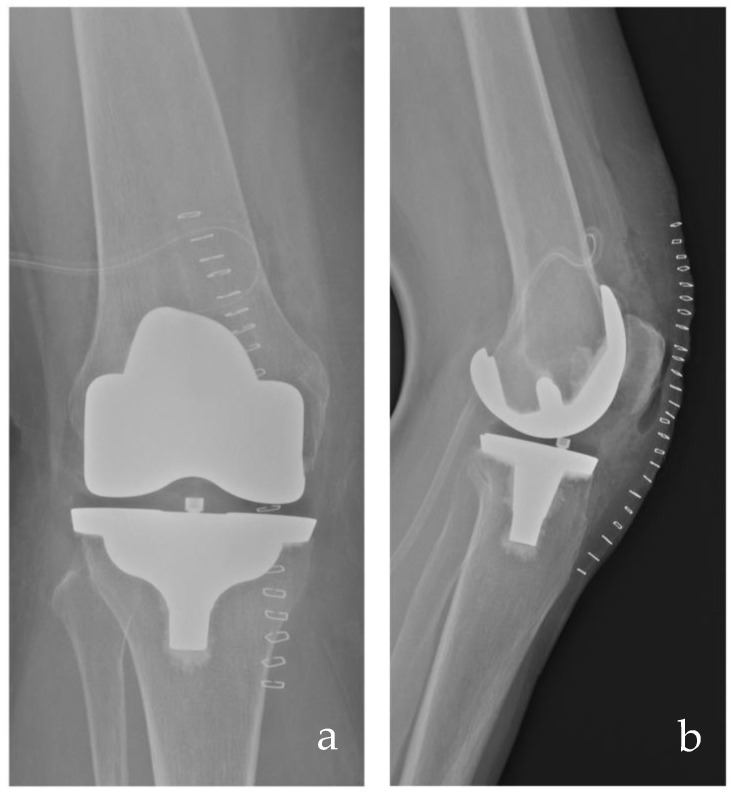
Post-operative anteroposterior (a) and lateral (b) radiographs of a medial pivot TKA from the senior author’s case series.
